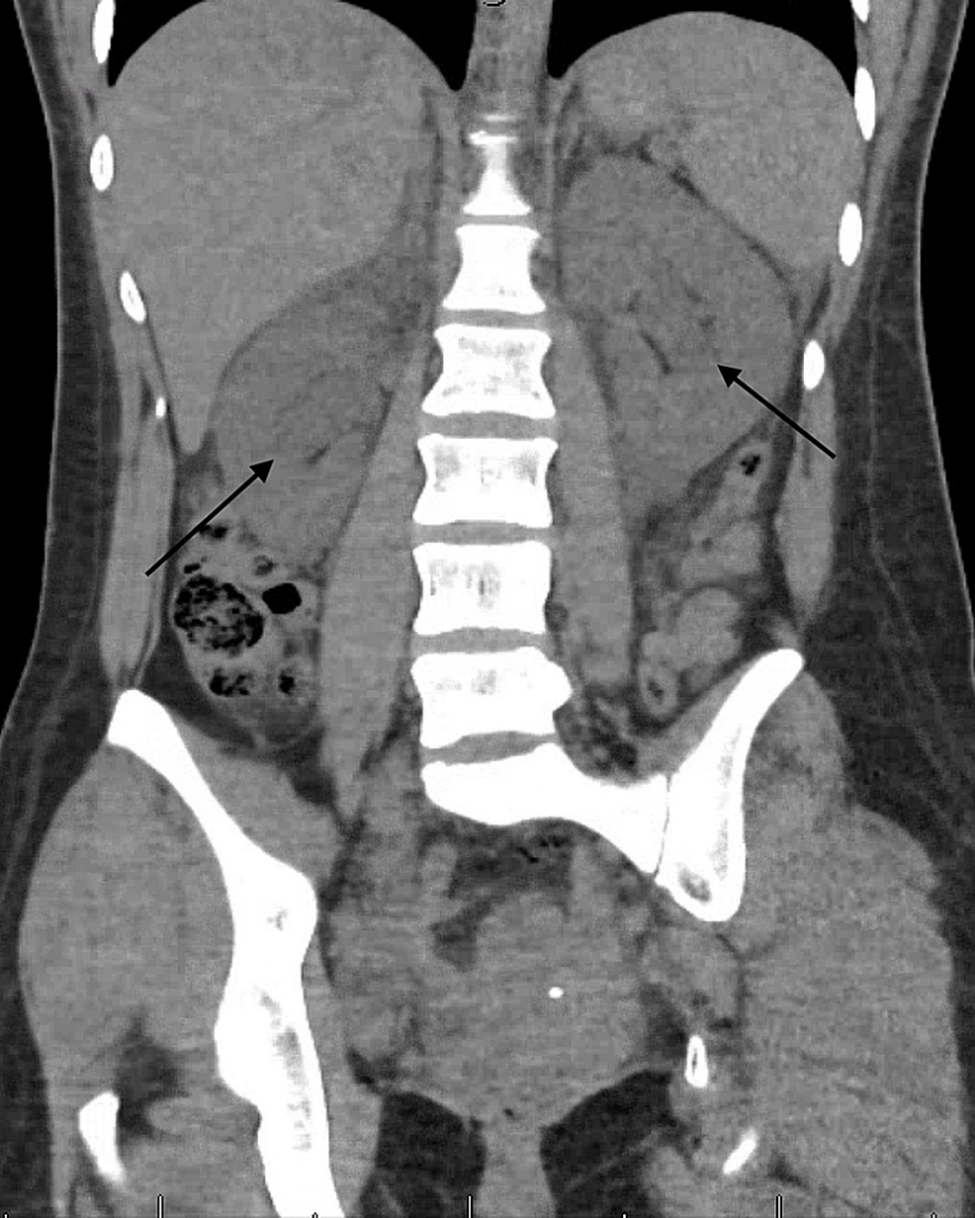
Coronal view of non-contrast CT abdomen pelvis showing bilateral hydronephrosis
